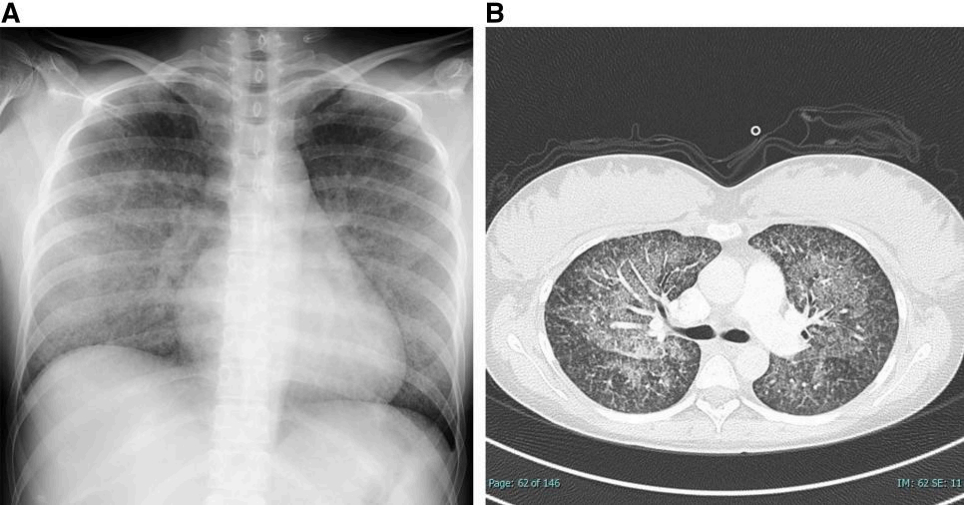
(A) CXR on day 6 (initiation day of steroid pulse therapy) revealed diffuse, homogenous, finely granular, cluster-like ground-glass opacities predominantly in the mid-to-lower lung fields. (B) CT scan on day 6. Extensive ground-glass opacities along with miliary shadows, consistent with ARDS secondary to miliary tuberculosis.
